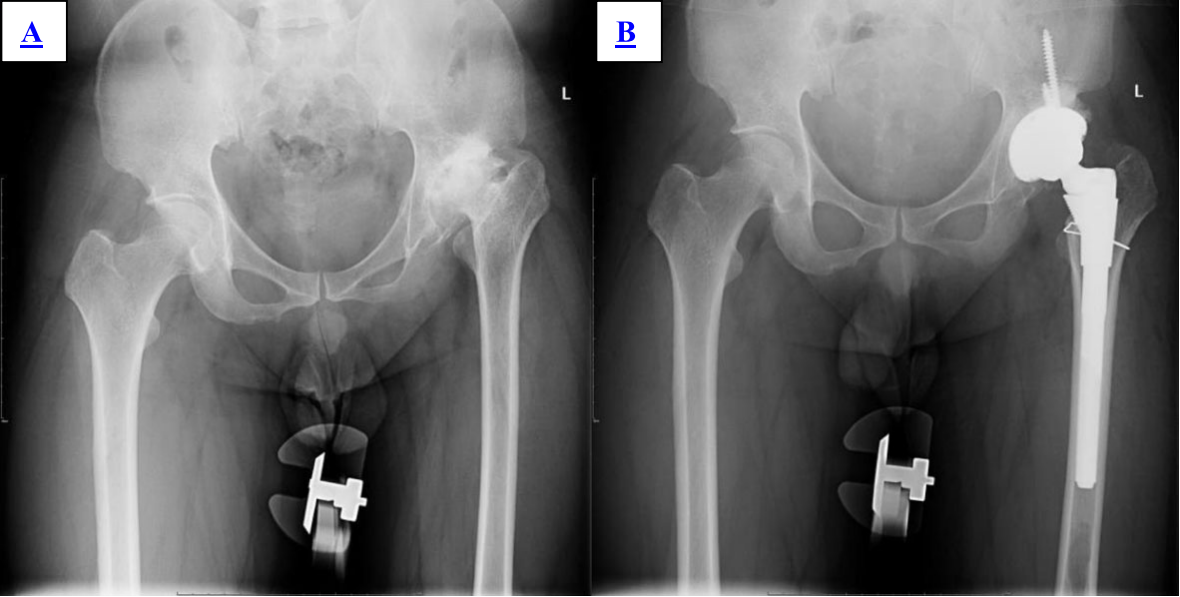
Male, 52 years old. Due to poor stability of LCU trial, the surgeon changed to S-ROM intraoperatively. a Preoperative X-ray showed left Crowe II DDH. b Postoperative X-ray
